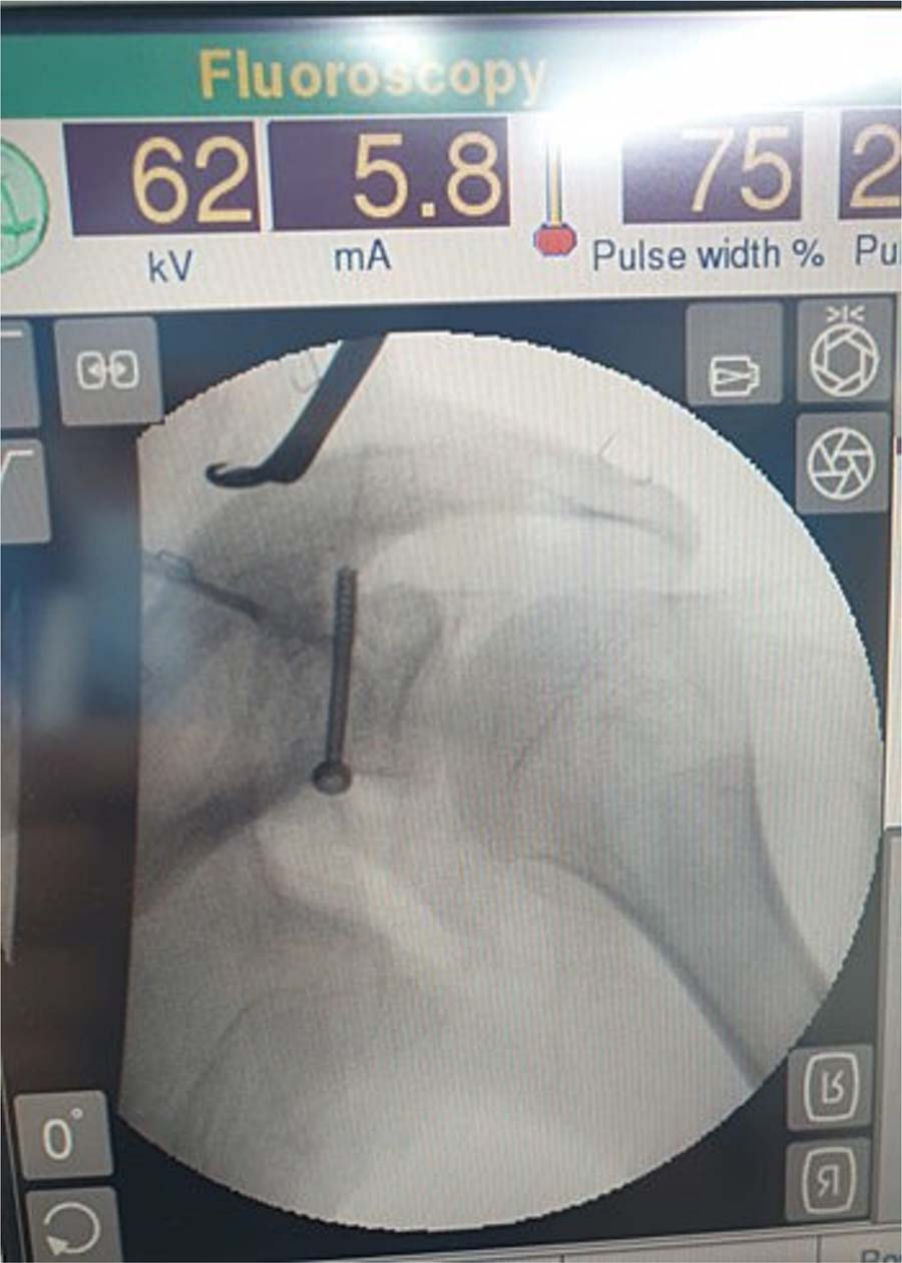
X-ray of the left shoulder post-operatively which shows proper placement of the screw.
